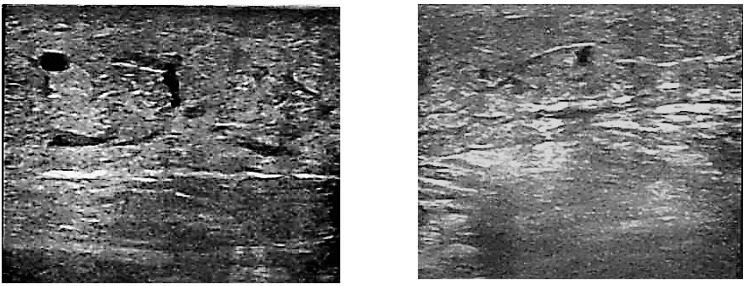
Ultrasound images of the perivascular space in a patient before (a) and after (b) 3 months of diosmin treatment.
